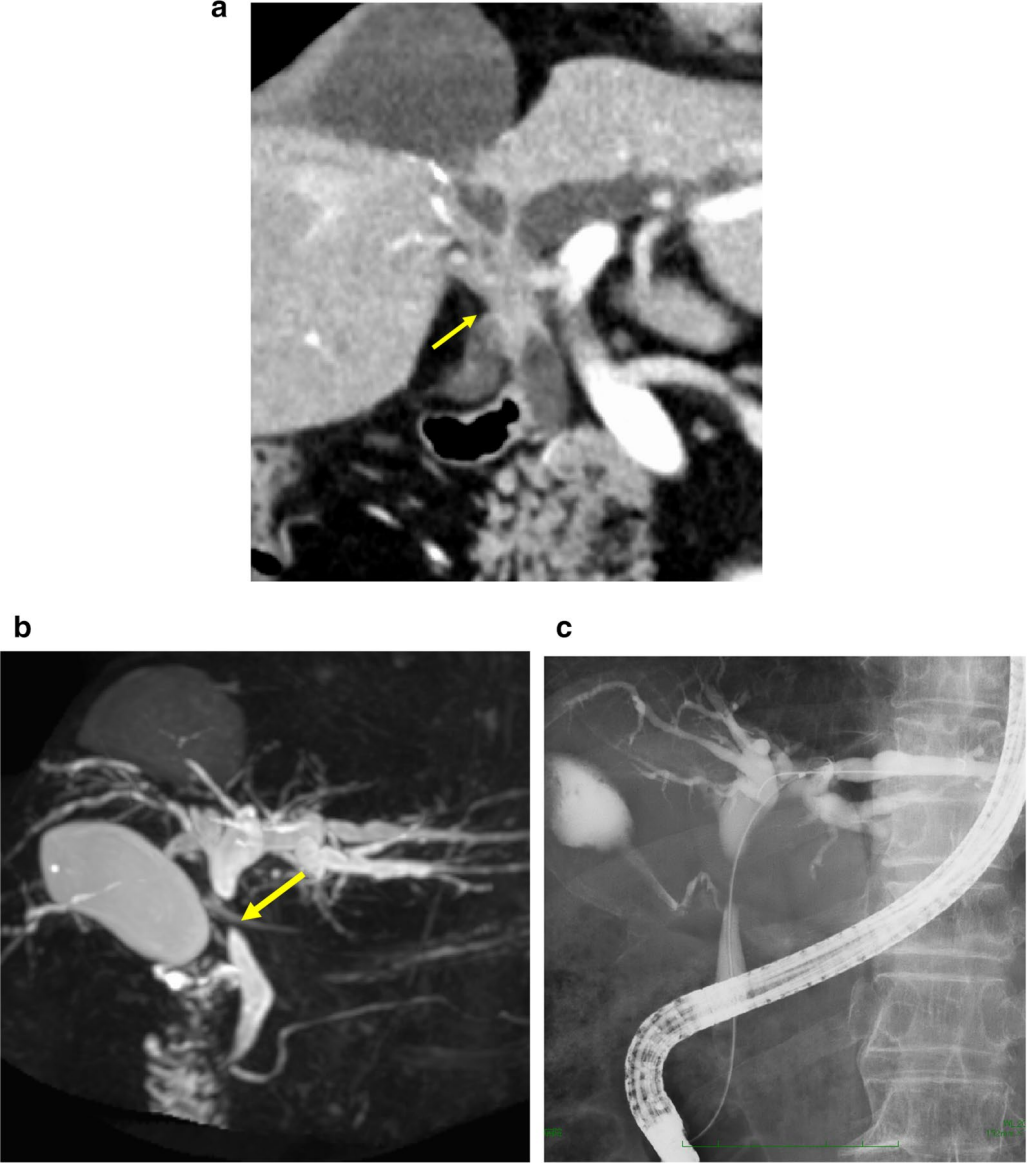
a Enhanced CT revealed an enhanced wall thickness in the hilar bile duct. b, c Magnetic resonance cholangiography, endoscopic retrograde cholangiography and intraductal ultrasound showed that the tumor had grown into the bile duct lumen
